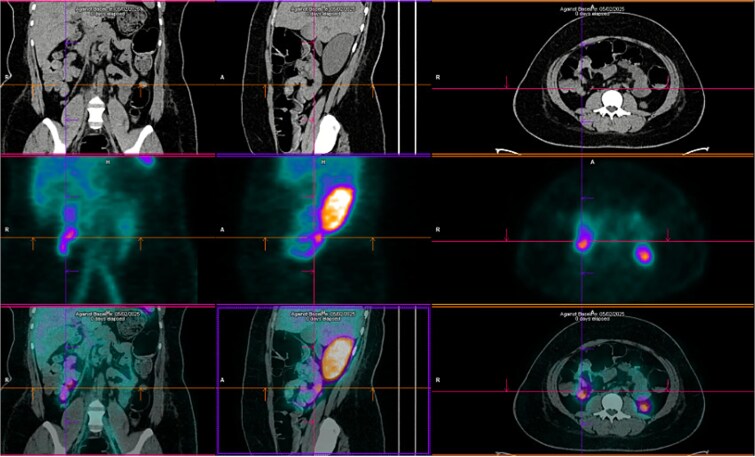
Technetium-labeled red blood cells scintigraphy showing two foci of active bleeding in the mesogastric and hypogastric regions.
